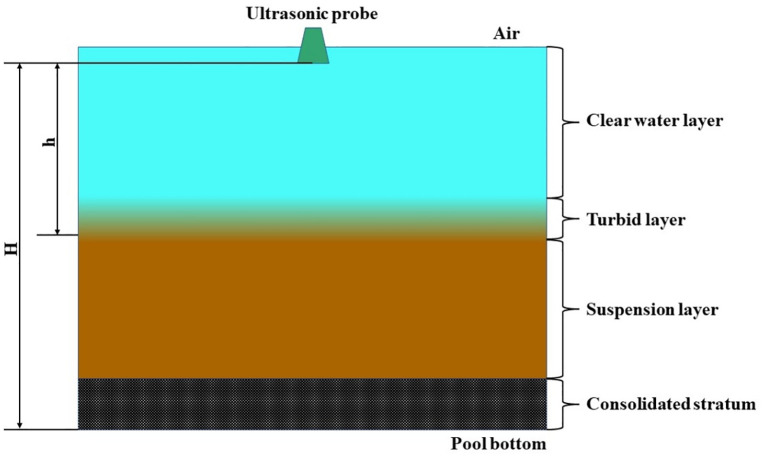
Ultrasonic probe installation and water layer.
